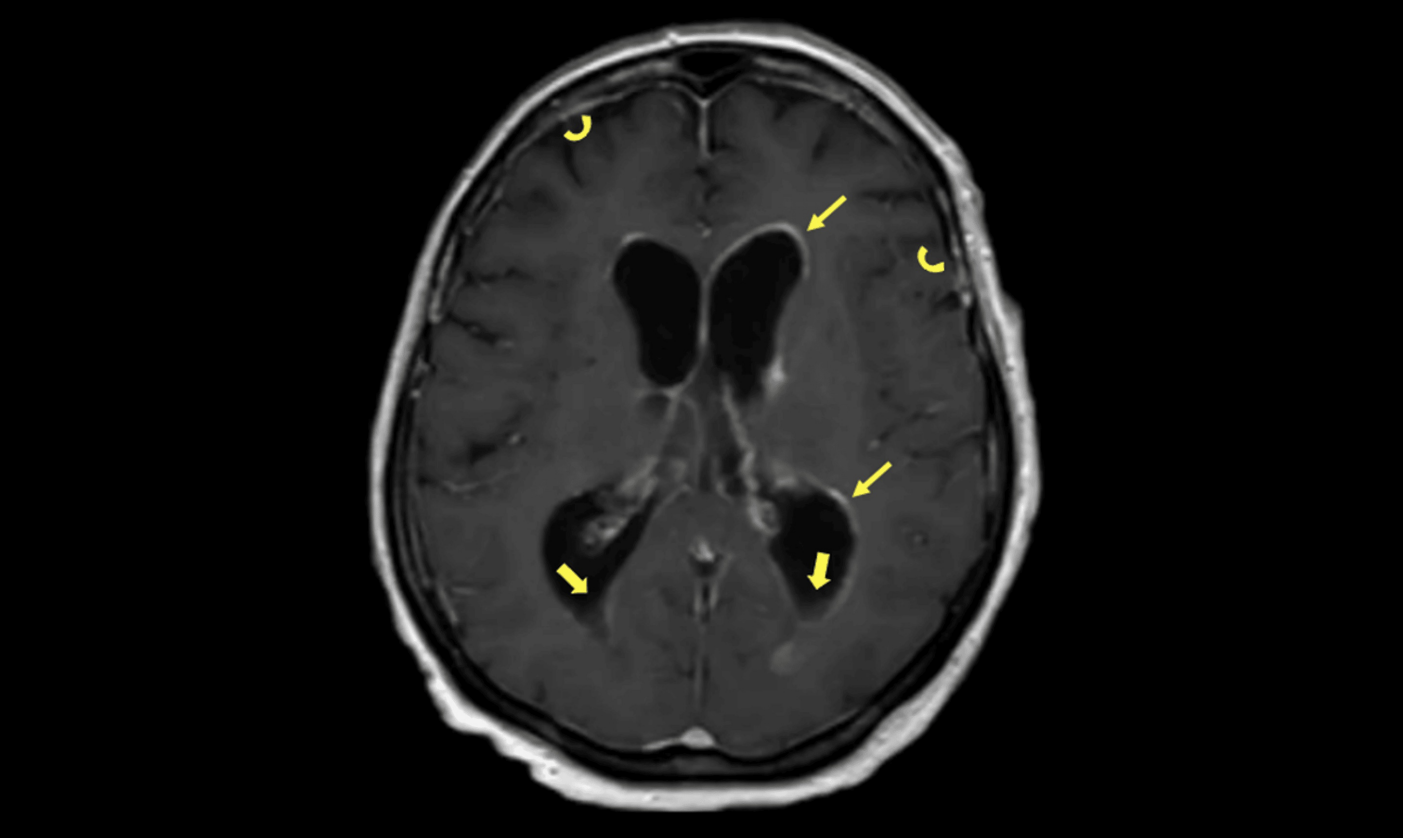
Post-contrast axial MRI of the brain. Demonstrates continued evidence of ventriculitis and meningitis: diffuse ependymal enhancement (thin yellow arrows), meningeal enhancement (curved yellow arrows), and layering debris ( thick yellow arrows).
